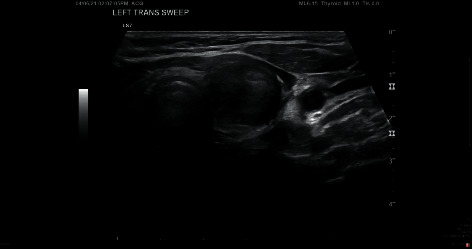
Ultrasound of the left thyroid lobe showing a heterogeneous mass.
